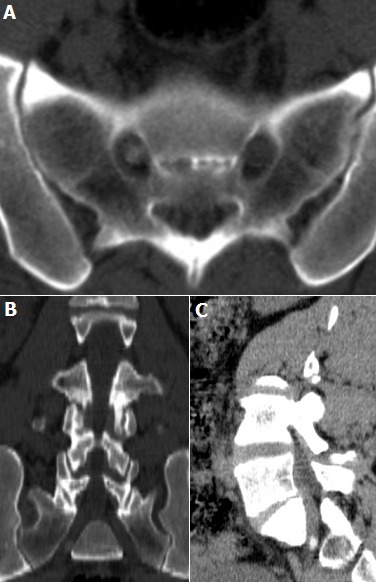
(A) axial CT slice presented in bone window: showed a round shaped calcification of the right S1 root; (B) coronal oblique reconstructions in bone window: showed a round shaped calcification of the right S1 root; (C) sagittal oblique reconstructions in soft tissue window: showed a round shaped calcification of the right S1 root
